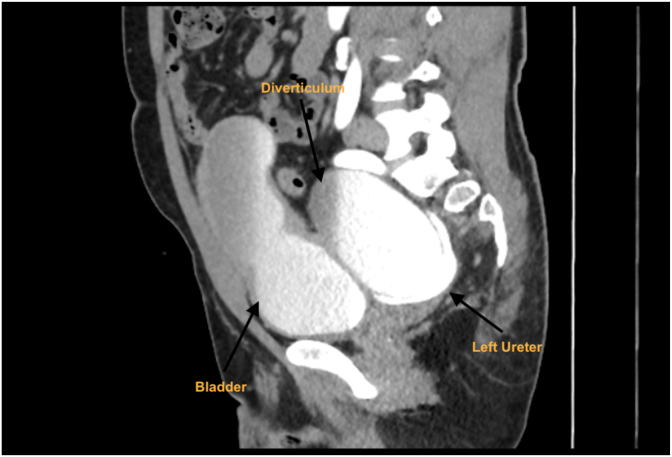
Computer tomography intravenous pyelogram (CT IVP) sagittal slice demonstrating direct compression of the left ureter by large bladder diverticulum.
